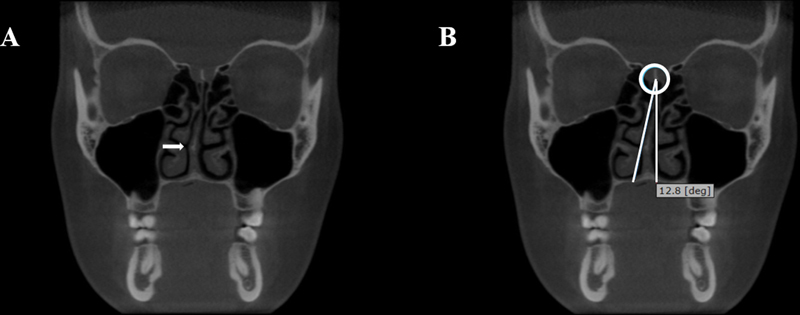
Coronal reconstructions of a CBCT exam showing a deviated nasal septum. (
